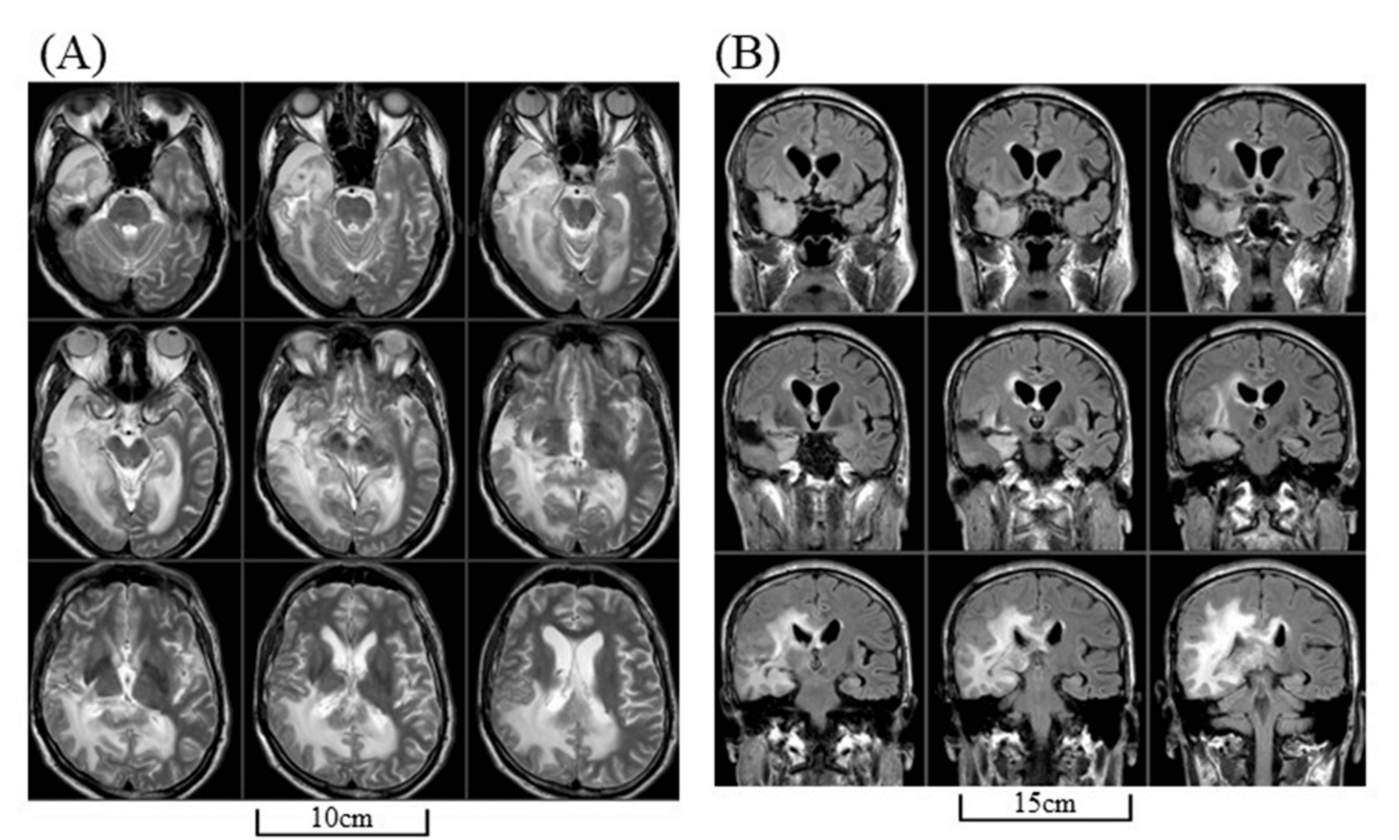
MRIs at recurrence (continued) The massive T2-high area extends even to the contralateral hemisphere by axial T2-weighted (A) and coronal FLAIR images (B). FLAIR: fluid-attenuated inversion recovery; MRI: magnetic resonance imaging
